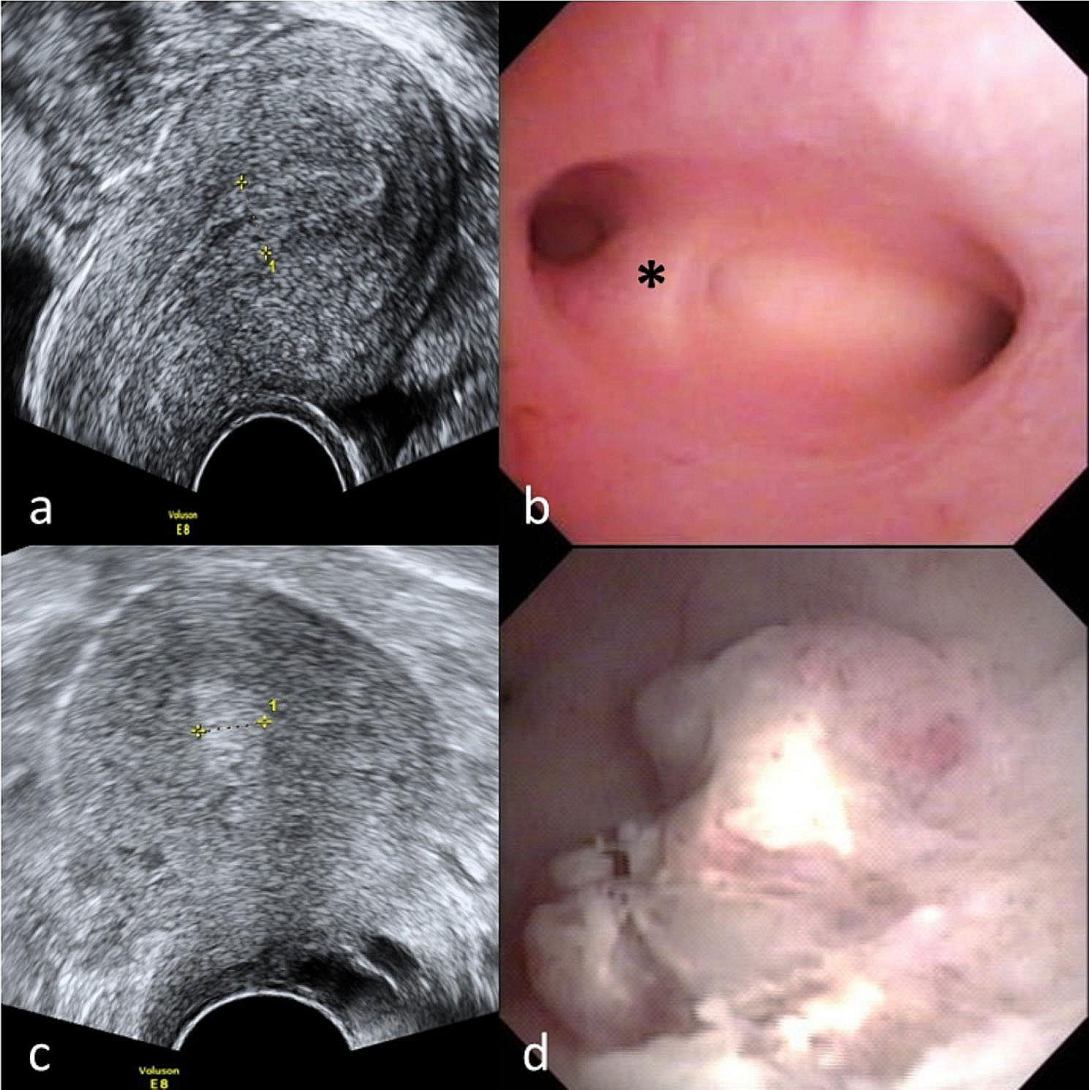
The transvaginal ultrasound and hysteroscopy finding with IUA (a&b) and RPOC (c&d). (a), A 34-year-old woman with G1P0AA1. The endometrial thickness was 0.79 cm. (b), The hysteroscopic image shows a dense IUA (asterisk) near the right ostium. The patient became pregnant during the follow-up period and did not receive a second hysteroscopy. (c), A 38-year-old woman with G1P0AA1. The endometrial thickness was 0.79 cm. (d), Hysteroscopy image revealing RPOC over the posterior cavity wall IUA, intrauterine adhesion; RPOC, retained products of conception
